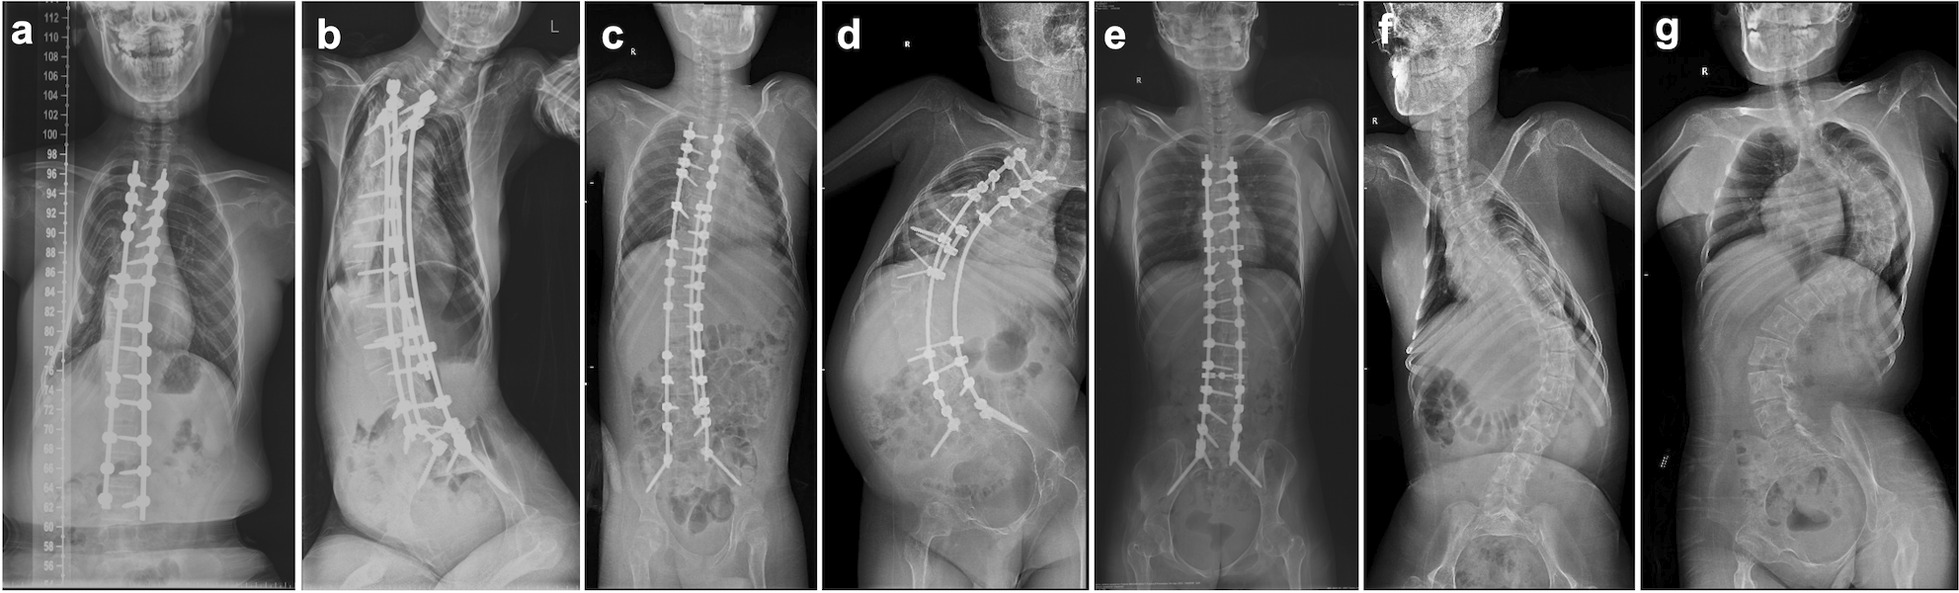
Anteroposterior full-body X-rays of SMA patients with spinal fusion or severe scoliosis. a–g were for cases 1–7, respectively
